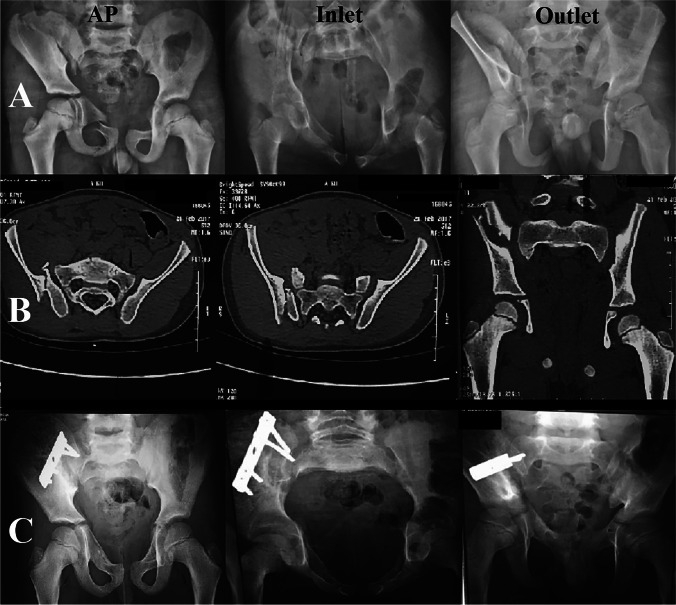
Male child 8 years old presented after fell from height with fracture pelvis type C 1.1 (vertical shear through Rt iliac bone, and Rt superior and inferior pubic rami) treated by posterior plating. Preoperative imaging: A plain radiographs and B CT scan. C plain radiographs after 14 months of follow-up
